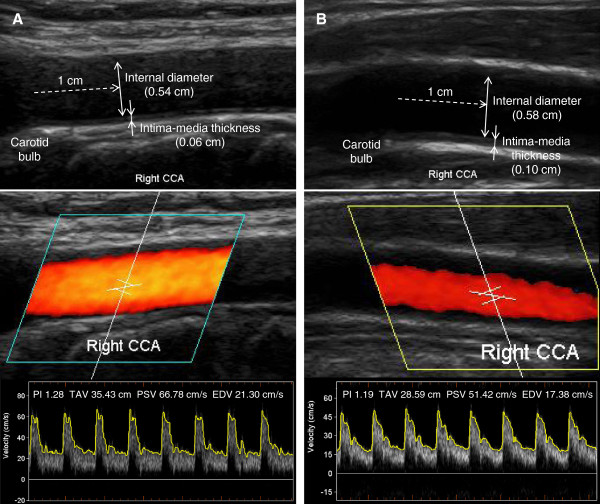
Representative ultrasonographic measurements of right common carotid internal diameters, intima-media thicknesses (IMTs), and blood flow velocities in a control subject (A) and a patient with lacunar infarction (B). Peak-systolic and end-diastolic internal diameters were measured at the segment 1 cm proximal to the beginning portion of carotid bulb, while IMT was measured at the peak of the R wave (end-diastole).
